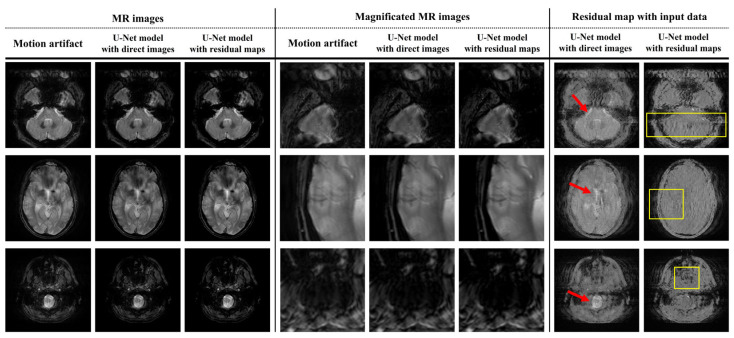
Results of applying U-Net models to real MR images with motion artifacts from ADNI database.
